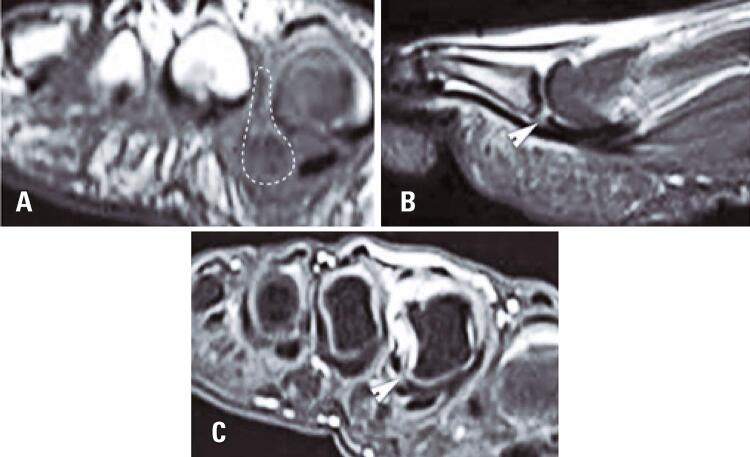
Sixty-two-year-old woman with complete plantar plate tear of the second toe. (A) short axis T1-weighted image with intermediate signal soft tissue (dotted line bordering) adjacent to the plantar plate of the second metatarsophalangeal joint and extends above the intermetatarsal ligament; (B) sagittal T2-weighted with suppression of fat sign image showing classic direct sign of plantar plate rupture with focal hypersignal separating the free margin of the plantar plate and the base of the proximal phalanx measuring 4mm (arrow);(C) Short axis T2-weighted with suppression of fat sign image showing focal discontinuity of the lateral margin of the 2nd plantar plate (triangle)
